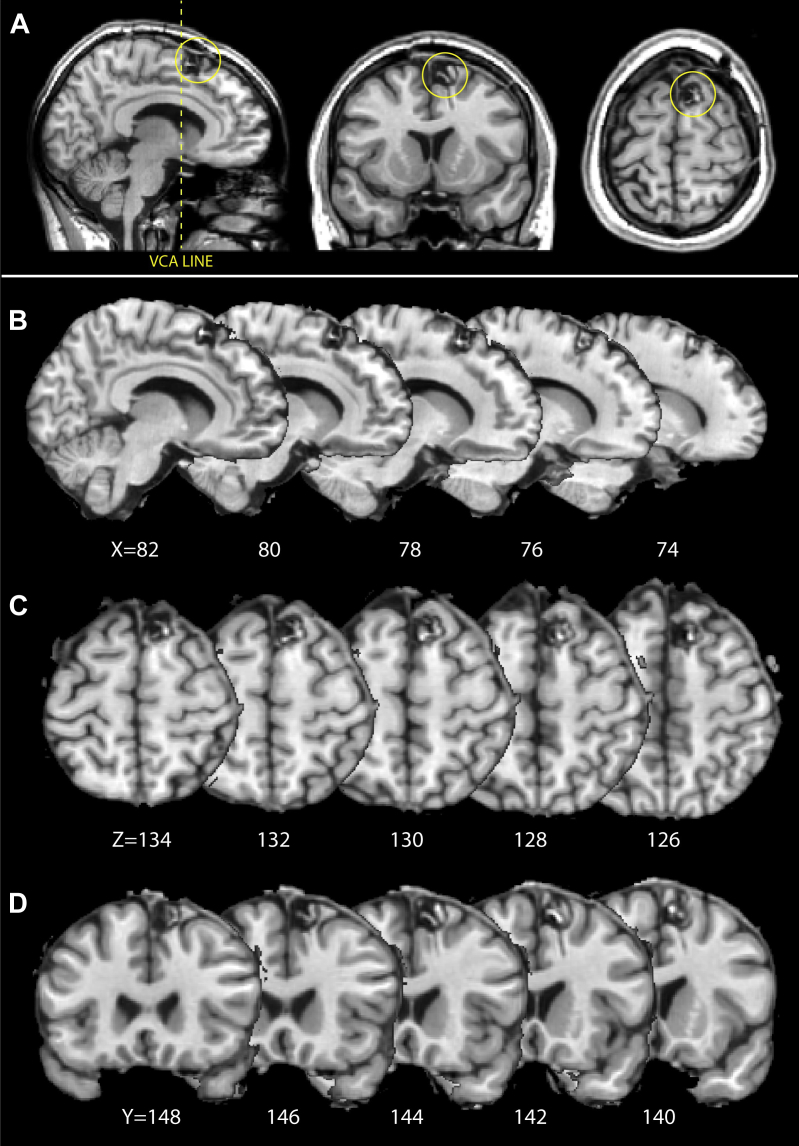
Lesion location. A) A high resolution T1-weighted MRI scan of patient KP in native space, the lesion location is circled in yellow. (B, C, D) The patient's brain image was normalised to standard MNI space and subsections show cross-sections for sagittal, axial and coronal sections, respectively. In panel B the VCA line is marked as a black vertical line, posterior to the lesion location. The lesion clearly lies medial to the superior frontal sulcus, anterior to paracentral sulcus, and dorsal to cingulate sulcus.
